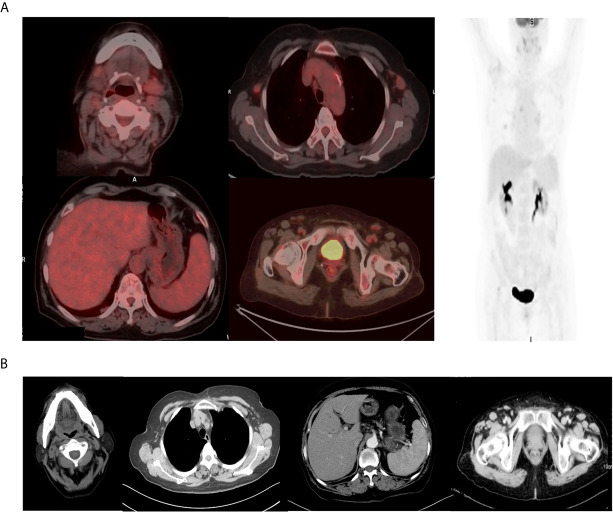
The final PET–CT and enhanced CT evaluation of the patient. (A) The final PET–CT evaluation showed complete metabolism remission after eight cycles of tislelizumab and lenalidomide. (B) The enhanced CT evaluation showed complete remission 4 months after PET scan.
